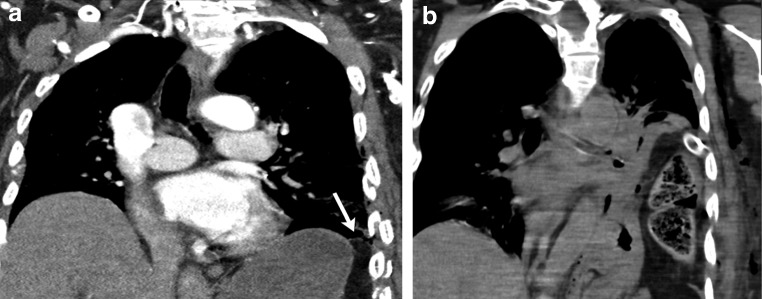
a, b An 80-year-old male with blunt thoracic and abdominal trauma following a motor vehicle accident. Coronal contrast CT (b) identifies multiple left rib fractures. In addition, there is a focal defect at the lateral aspect of the left hemidiaphragm (white arrow). The patient presented 2 days later with increasing shortness of breath and a new opacity on the frontal radiograph. Coronal image from follow-up CT (b) identifies interval enlargement of the left lateral diaphragmatic defect with new herniation of the stomach, splenic flexure and omentum into the left thorax. Emergent surgical repair was performed for this hernia
